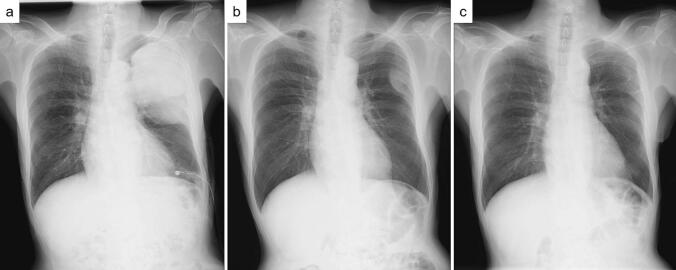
Chest radiography. (a) Preoperative chest radiograph showing a large mass in the left thorax measuring 135 × 80 mm after pleural effusion drainage. (b) A chest radiograph taken at the time of cataract surgery 4 years prior revealed a mass measuring 43 × 22 mm. (c) Follow up chest radiography at the time of 1 year and three months after surgery.
